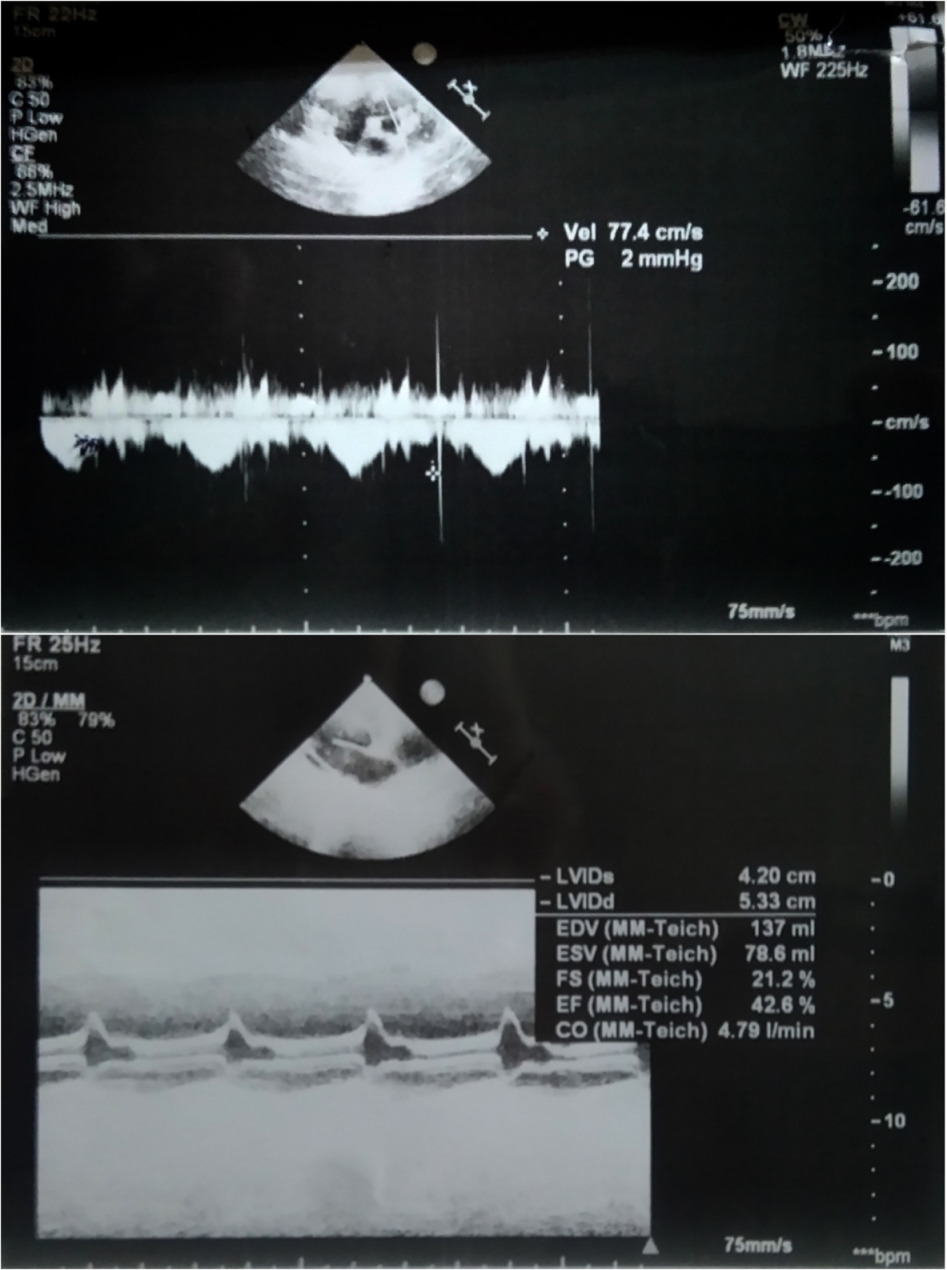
Parasternal long-axis transthoracic echocardiogram 1 week after initiating treatment shows minimal improvement in cardiac function
